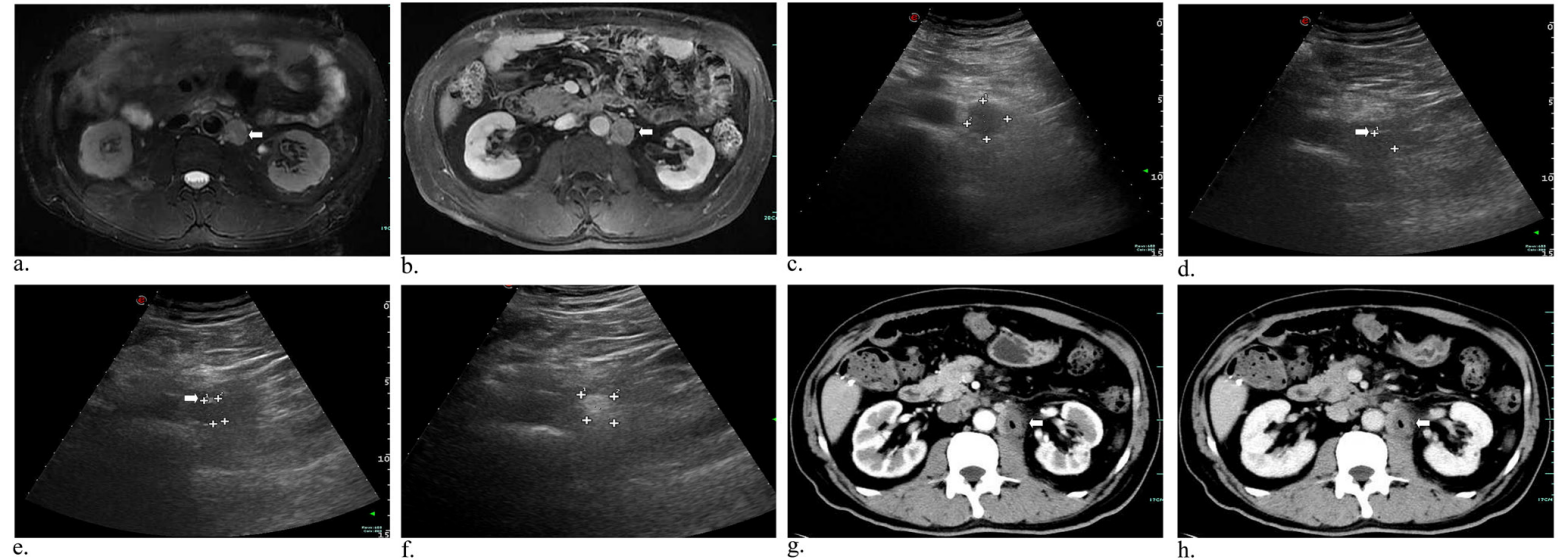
Metastasis of lymph nodes in a 60-year-old man who had undergone gallbladder cancer resection MR image of T2-weighted and substance phase showed enlarged, round lymphoma around retroperitoneal abdominal aorta a.,b. (arrowhead). Preoperative US image revealed a hypoechoic mass 2.52*2.26 cm c.. Intraoperative sonogram showed the one d. (arrowhead) and two needles e. (arrowhead) inserting into the tumor, suggesting the distances (cm) of needle tip to tumor margin (anterior: 1.61; 1.54). Afterwards, the mass had local enhancement under ultrasound scanning f., and increased unenhanced low-density areas in arterial g. (arrowhead) and substance phase h. (arrowhead) on contrast-enhanced CT scan.
